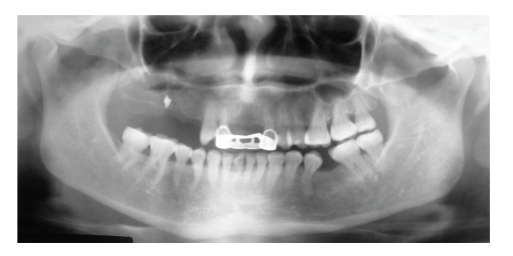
Panoramic X-ray. Notice the presence of a right maxillary radiopacity and the absence of teeth 1, 2, 3, 4, and 5, all with adequate bone healing except the 3 area.
